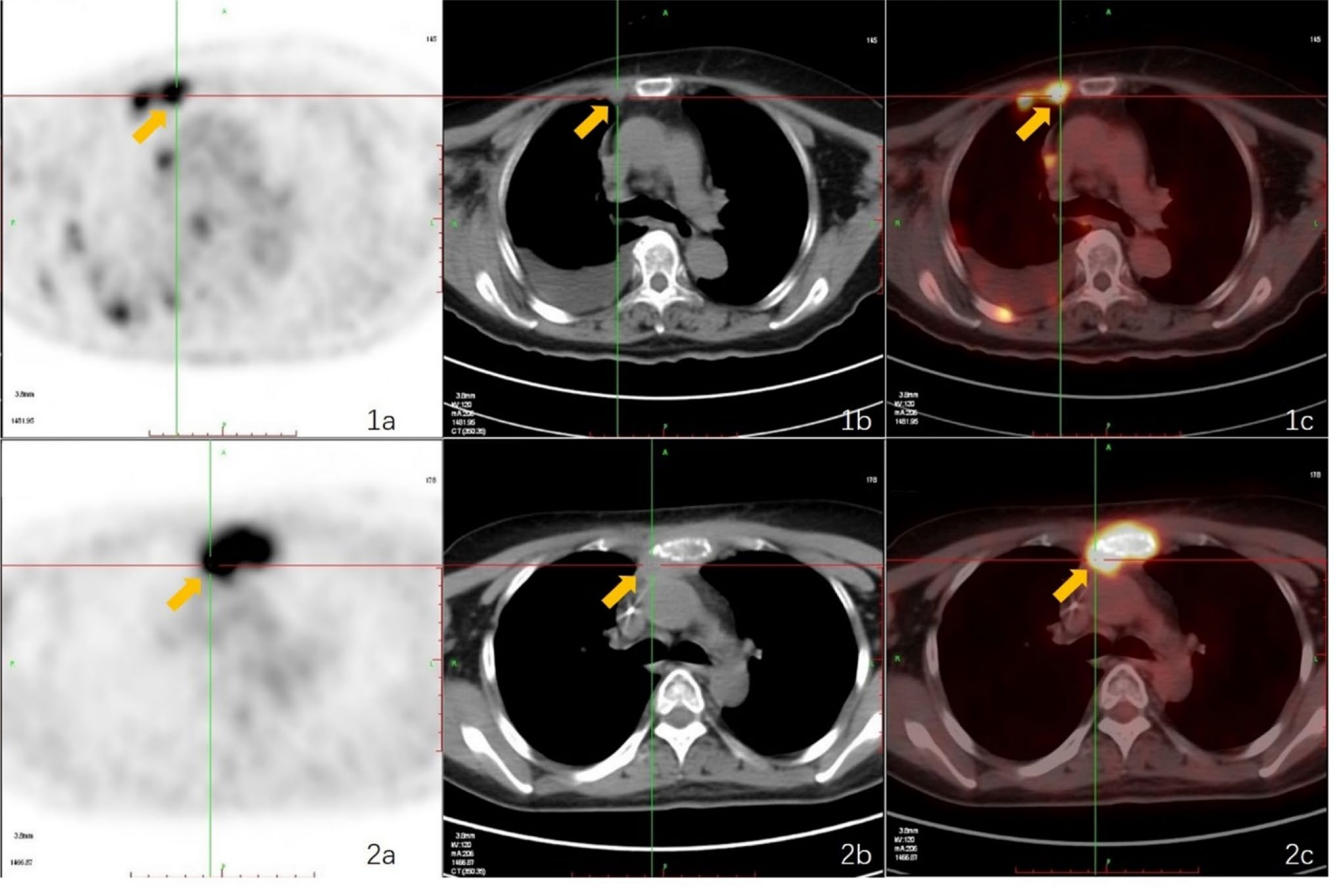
Internal mammary lymph node metastasis (yellow arrow) with sternal erosion detected based on FDG uptake (1a), computed tomography (1b), and a fused FDG PET/CT image (1c), as well as sternal metastasis (yellow arrow) with a local soft tissue lump detected based on FDG uptake (2a), computed tomography (2b) and a fused FDG PET/CT image (2c)
